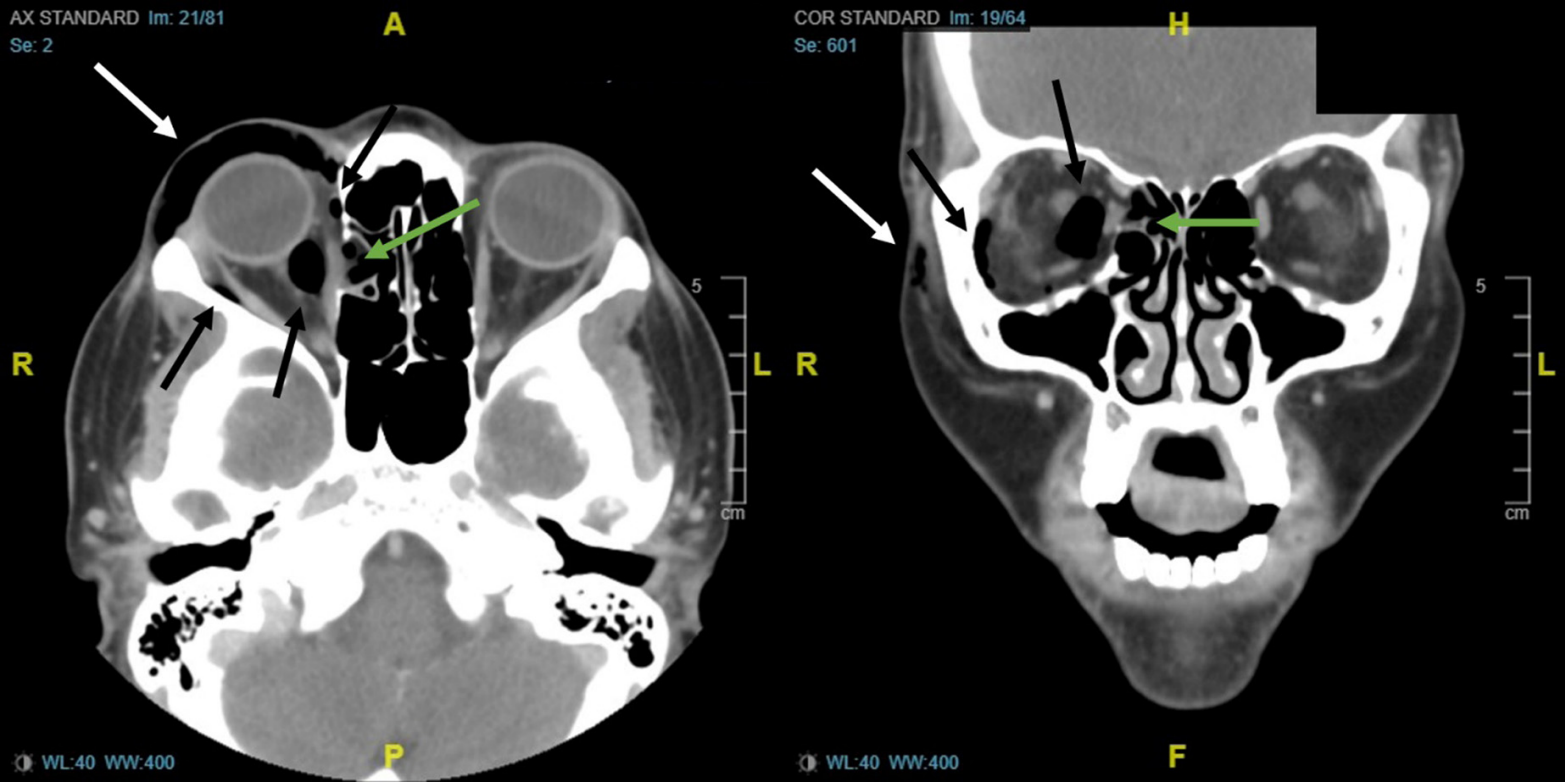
Computed tomography of the facial bones, axial view on the left and coronal view on the right, demonstrating subcutaneous periorbital emphysema (white arrows), intraconal orbital space emphysema (black arrows), with a defect in the lamina papyracea of the ethmoid sinus (green arrows).
